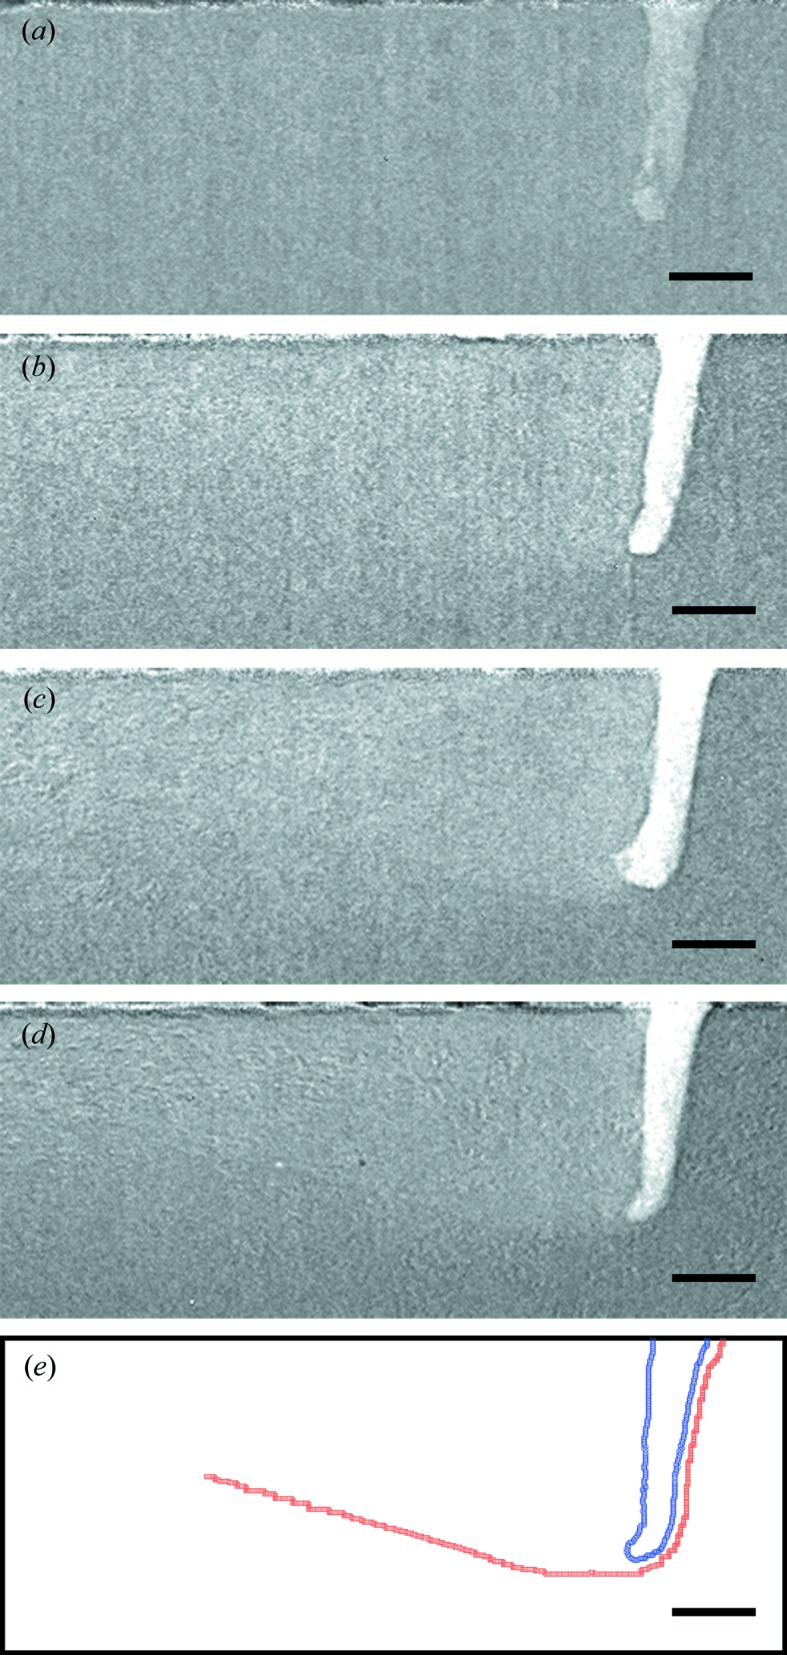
Influence of camera exposure time on imaging of the solid–liquid interface during the laser AM process. (*a*) 1 µs. (*b*) 5 µs. (*c*) 10 µs. (*d*) 20 µs. (*e*) The solid–liquid interface (red squares) and liquid–gas interface (blue circles). The laser was scanned from left to right in this and all experiments. The vapor hole (depression) is comparable in diameter with that of the laser beam at the top surface of the metal. The thickness of the liquid layer in front of the vapor depression is small, whereas the melt pool extends several hundred micrometres behind the depression. The sample thickness (along the X-ray beam direction) is 380 µm. The laser power was 260 W and the scanning speed was 500 mm s^−1^. The scale bars are 100 µm.
